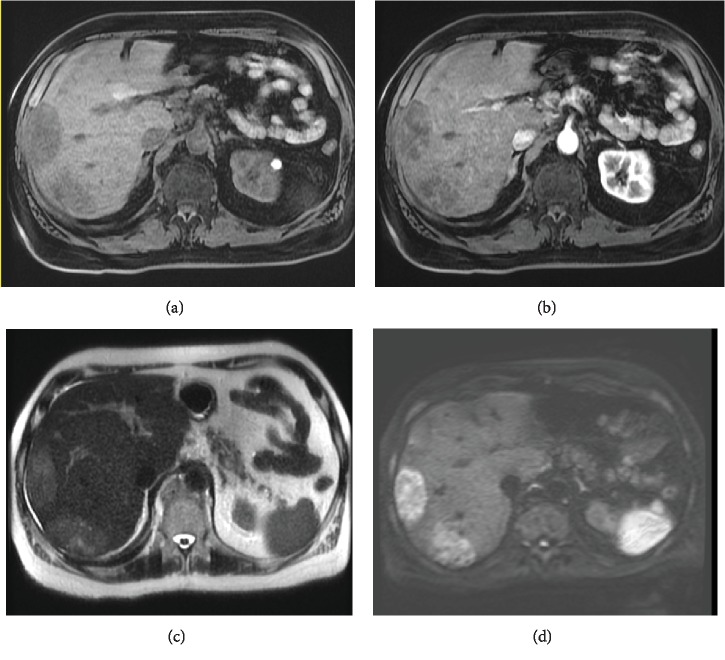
Hepatic metastases on MRI. (a) Axial T1-weighted fat-suppressed precontrast image and (b) postcontrast arterial phase image demonstrating multiple right hepatic lesions with internal enhancement. (c) Axial T2-weighted image shows mildly high signal within the hepatic lesions. (d) Axial diffusion weighted image (*B* = 800) with high signal within the right hepatic lesions compatible with restricted diffusion.
